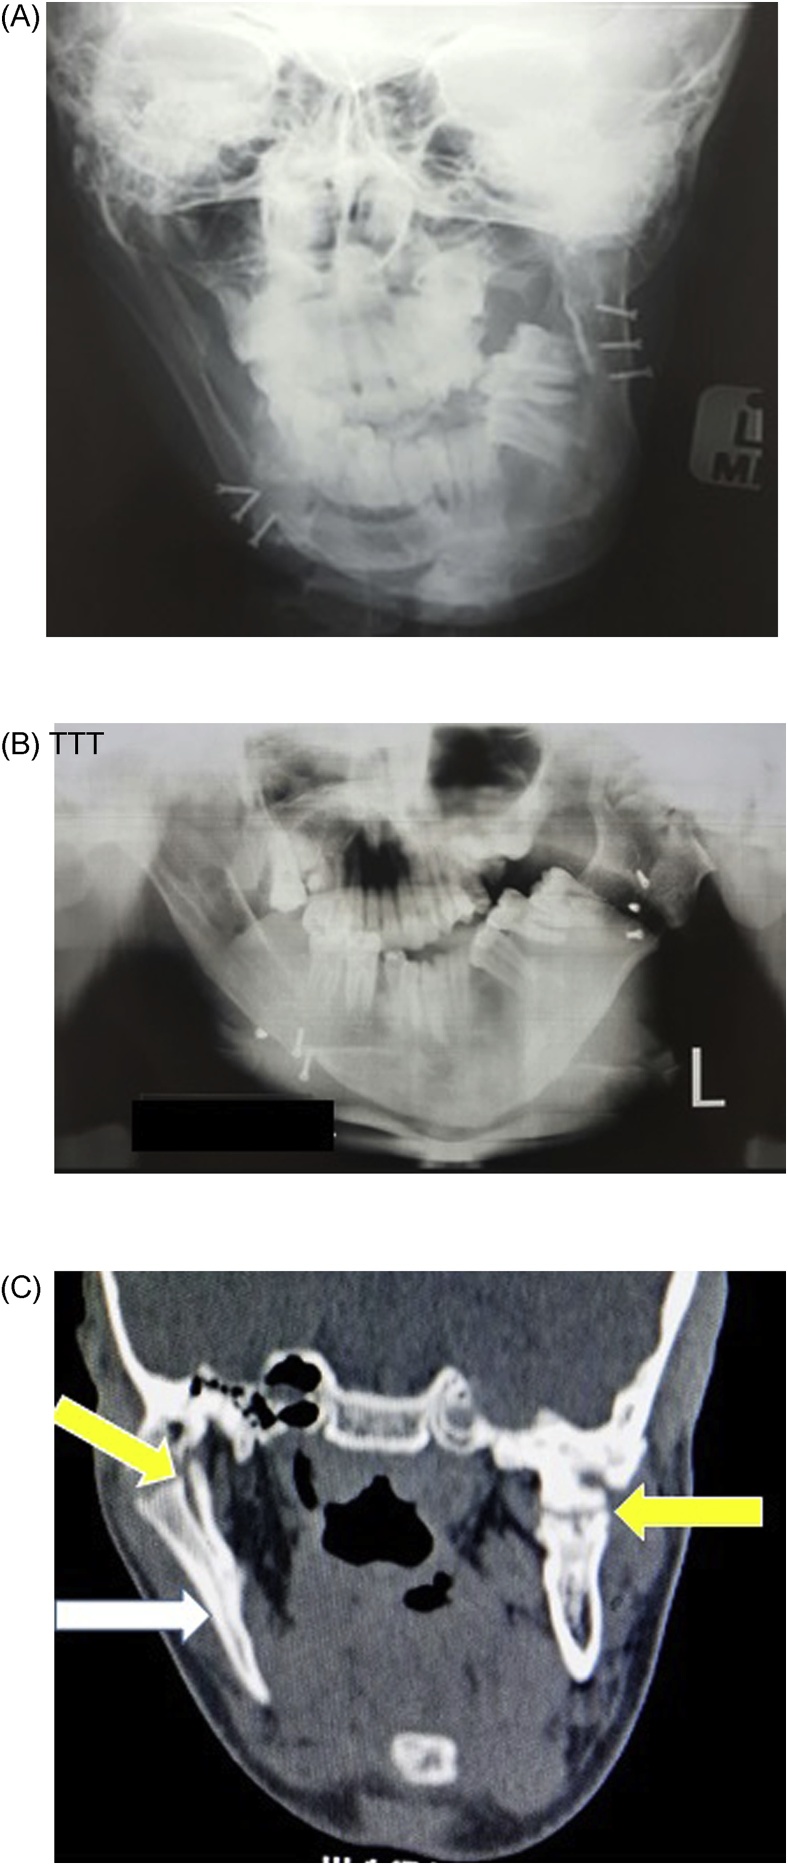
(A) Pre-operative radiographs at age 16 years: postero-anterior (mandible). (B) Orthopantomography. (C) Coronal CT scan.
